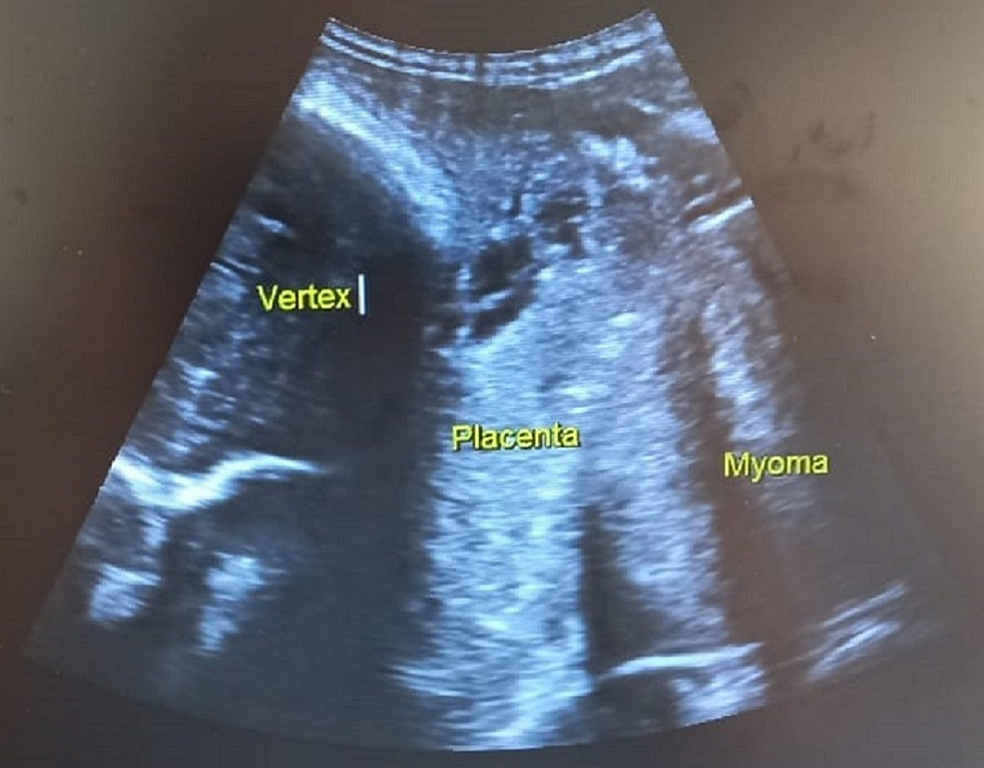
Abdominal ultrasound image showing the head of the fetus, central placenta previa, and the cervical fibroid or myoma
